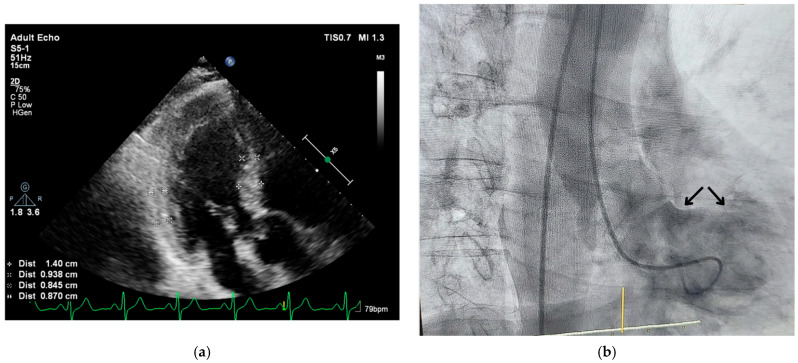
(a) A two-dimensional echocardiographic image of a hypertensive patient with a sigmoid septum and ampulla-shaped LV cavity geometry during diastole. Reprinted from ref [9]. (b) Preexisting Triple S in the same patient, similar to Takotsubo geometry. Reprinted from ref [9].
